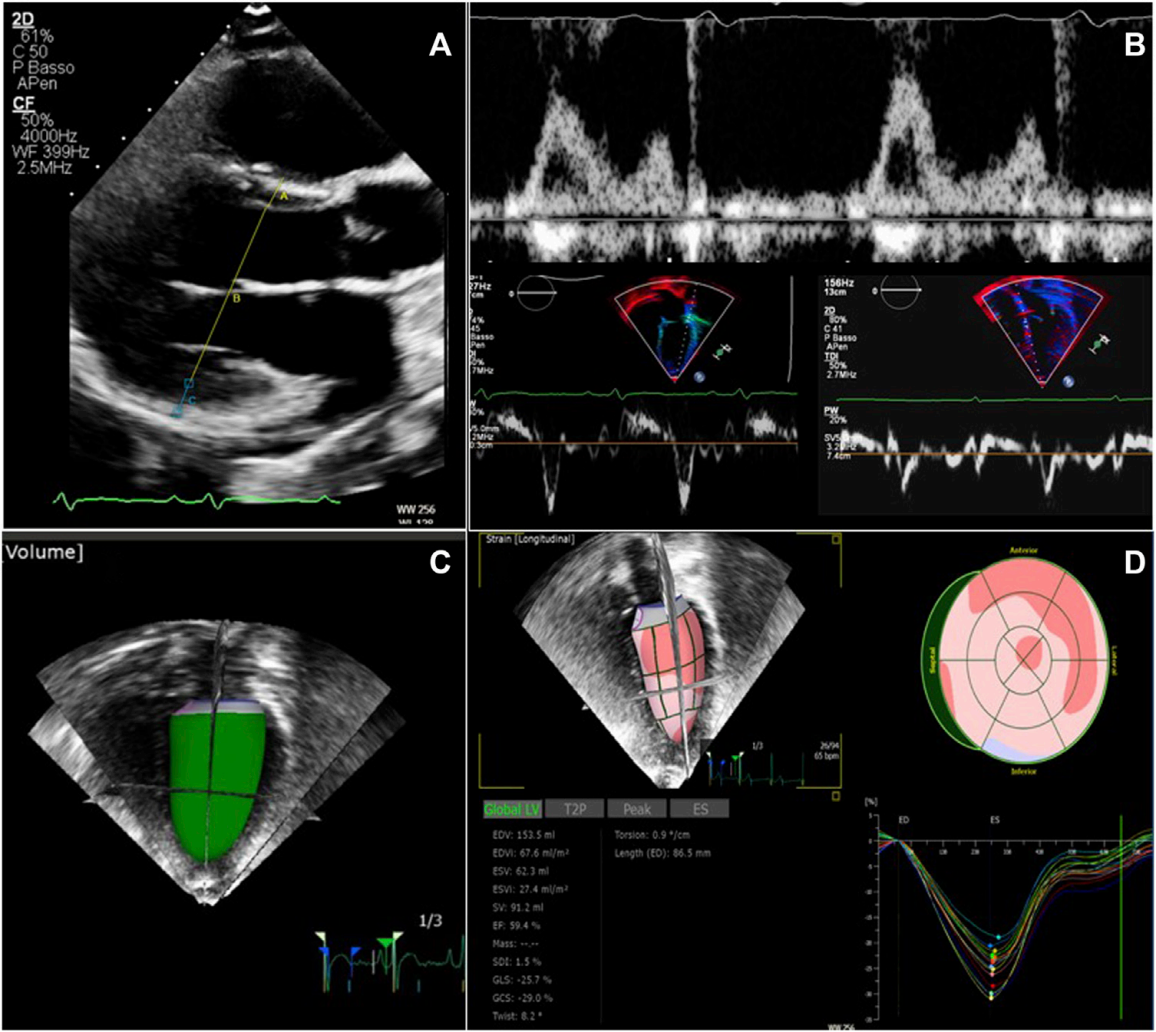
Echocardiographic examination in patients with ADPKD and ARPKD. (A) Left ventricular mass calculation from two-dimensional parasternal long axis view in end-diastole. (B) Diastolic function evaluation merging data from transmitral inflow velocities (upper image) and tissue Doppler velocities (lower images) from both the lateral and the septal left ventricular wall. (C) Real time three-dimensional left ventricular volume estimate (D) Global longitudinal strain analysis from speckle tracking imaging, using real time three dimensional acquisition.
